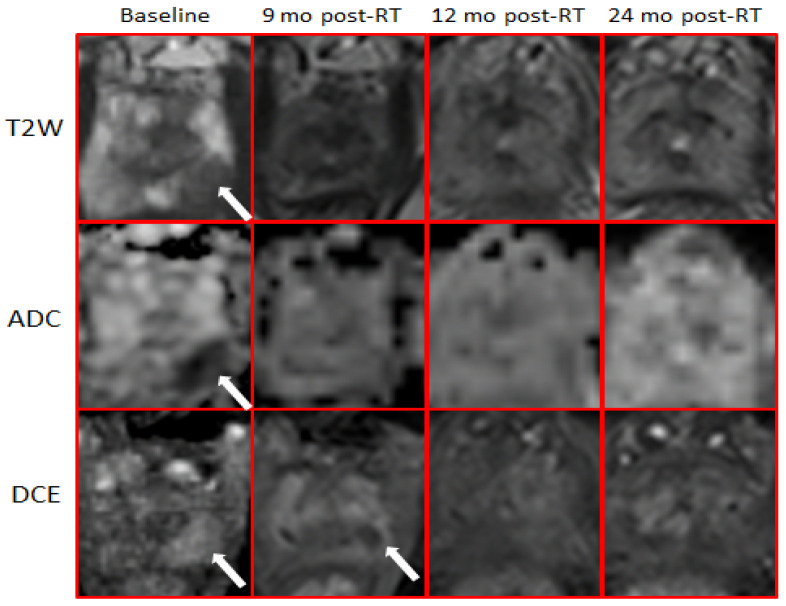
Longitudinal changes of mpMRI following RT. T2-weighted, ADC, and early enhancing images from the DCE-MRI series are shown. At baseline, the arrows indicate the GTV, defined by hypointensity on T2-weighted, ADC, and enhancing on DCE. There is no hyperintensities on T2-weighted and ADC post-RT. DCE at 3-months post-RT indicates complete obliteration of the GTV (white arrow).
